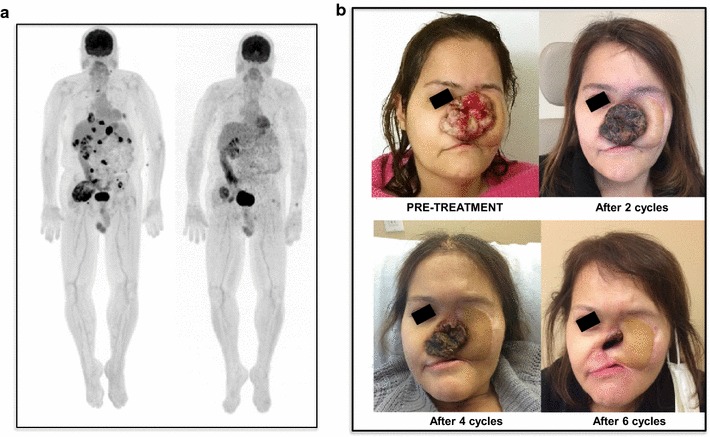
Partial response (PR) to nivolumab in 2 patients. a PET/CT of a 74 year-old male with metastatic dedifferentiated chondrosarcoma after six cycles of nivolumab alone; he is maintaining a PR after 26 cycles. b 46 years-old woman with osteosarcoma, treated with nivolumab for six cycles; pazopanib 800 mg p.o. daily was started after 4 cycles of nivolumab. She underwent resection with negative margins
